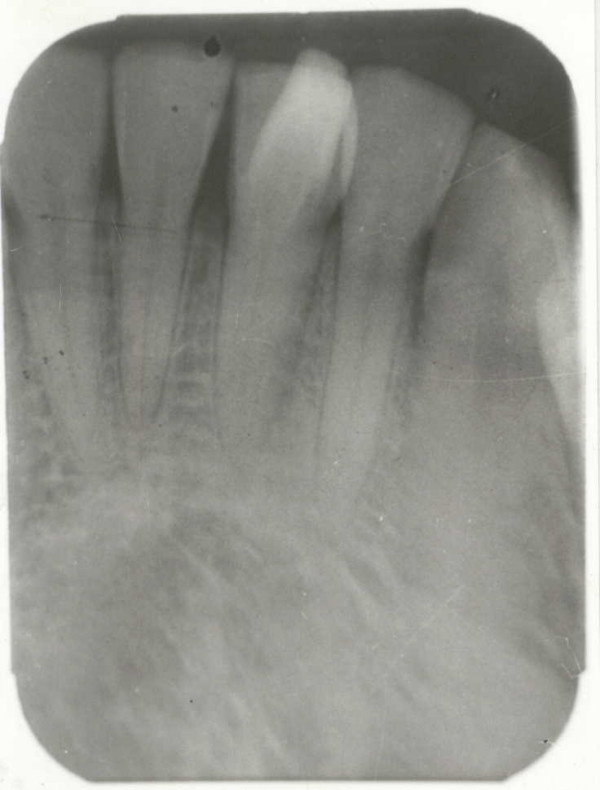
Peri-apical radiograph of the mandibular left central incisor showing the inverted V-shaped talon cusp.
